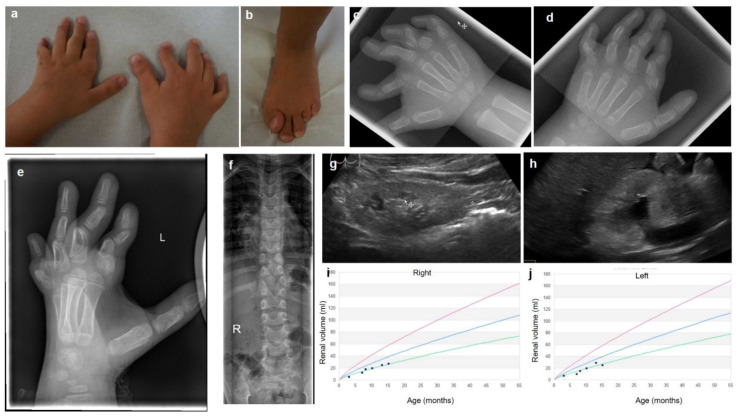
Phenotypic features resulting from KYNU loss of function. Characteristic hand and foot malformations (a–e), including hyperphalangism (c–e), lumbosacral wedge vertebrae (f) and bilateral renal hypodysplasia (ultrasound images, g,h; renal volumes depicted in i,j).
